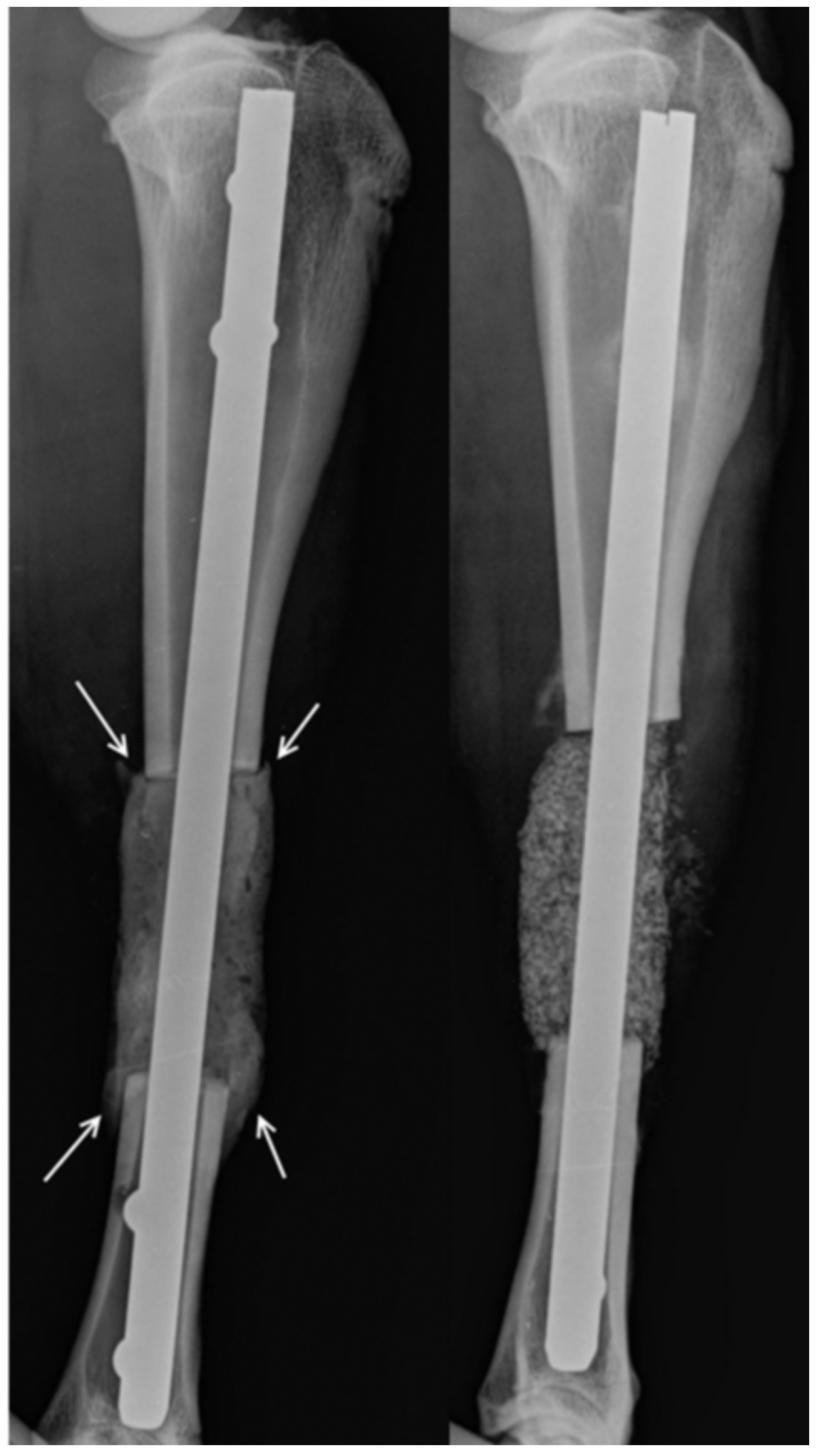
Post-operative radiographs showing PMMA spacer with overlapping edges after the first surgery on the left and the graft filled capsule following the second surgery on the right.
